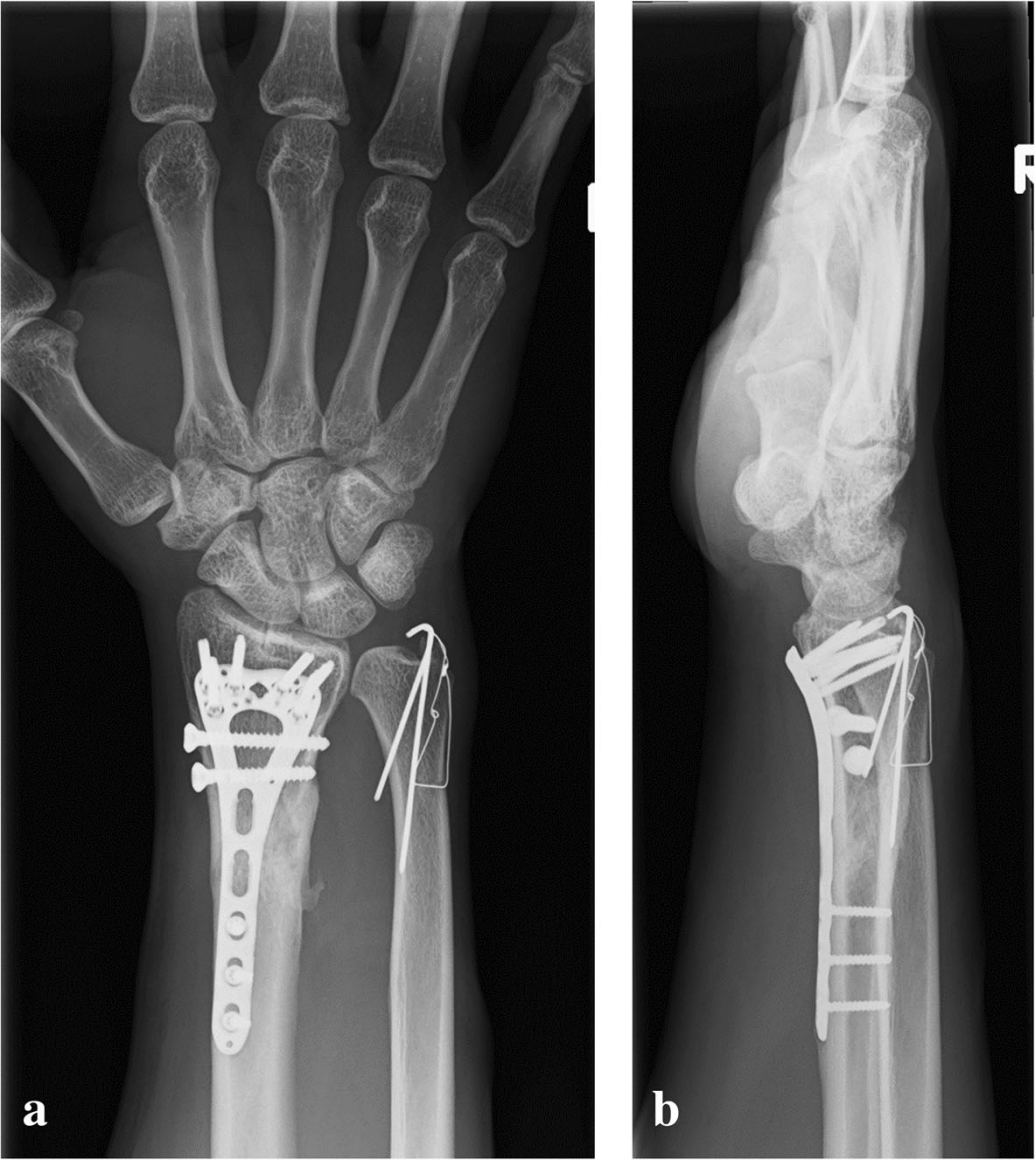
Plain radiograph of the right wrist at 6 months postoperatively. (a) Anteroposterior view and (b) lateral view. Bone union of the radius and ulna is achieved
